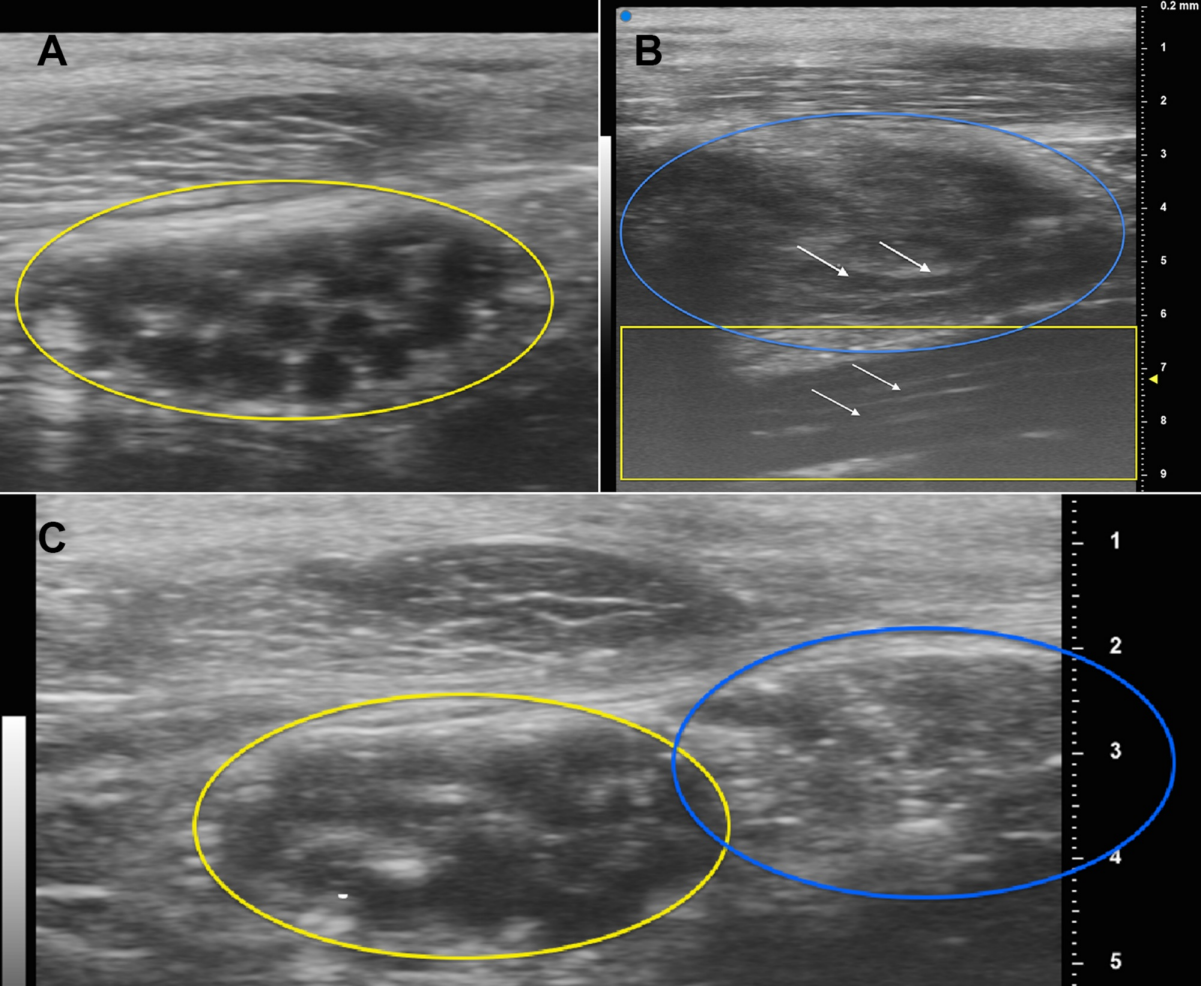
Ultra-high-frequency Ultrasound Images A) Cross-section of the median nerve distal to the neuroma (yellow oval); B) long axis of the neuroma (blue oval) and median nerve (yellow box); white arrows point to the long axis of the median nerve fascicles; C) cross-section of the median nerve (yellow oval) and neuroma (blue oval).
